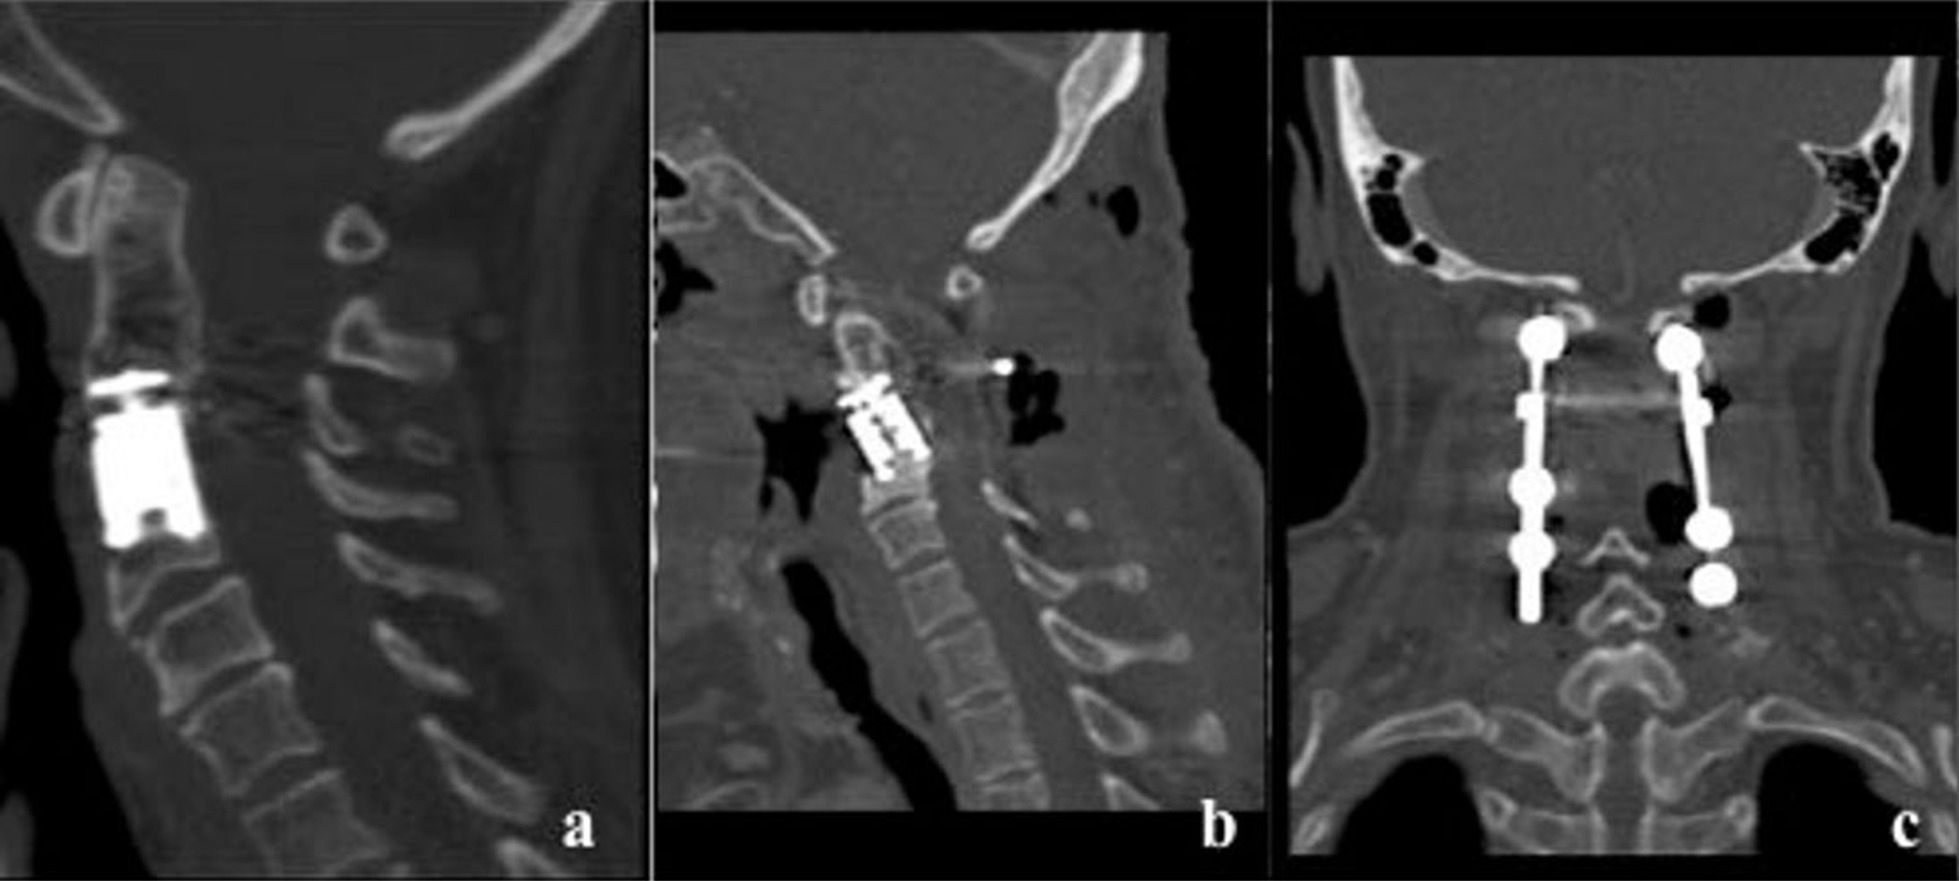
a Computed tomography (CT) image after the first surgery shows well-aligned C3 interbody expandable device; b*, *c CT images after the last surgical procedure show partial interbody device failure and posterior cervical stabilization
